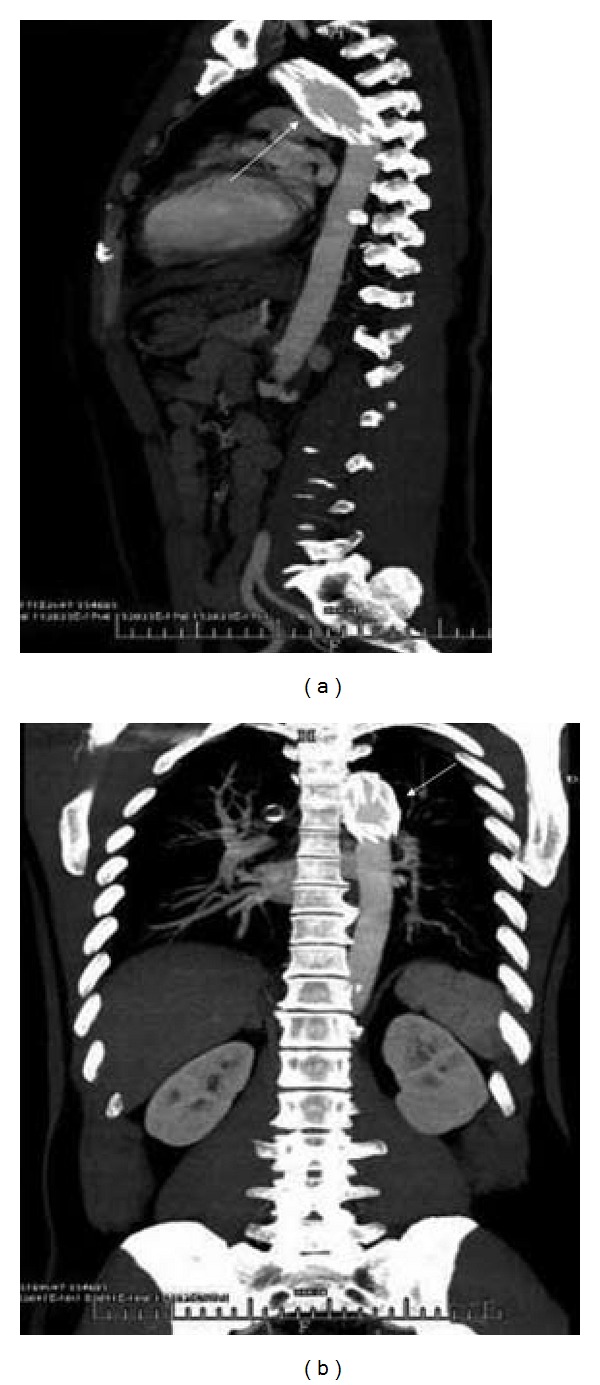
The CTA 10 years after endovascular treatment: the endograft remained in the original position with slight dilation at its center (arrow).
